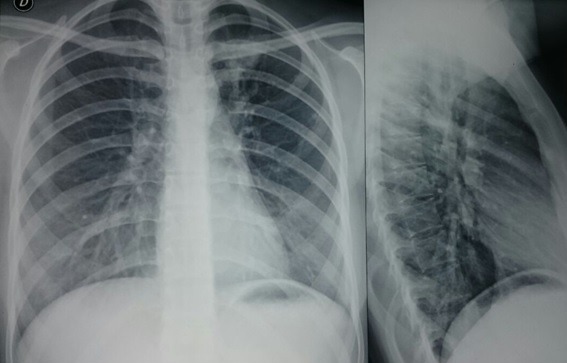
Front to back and lateral chest x-rays.
